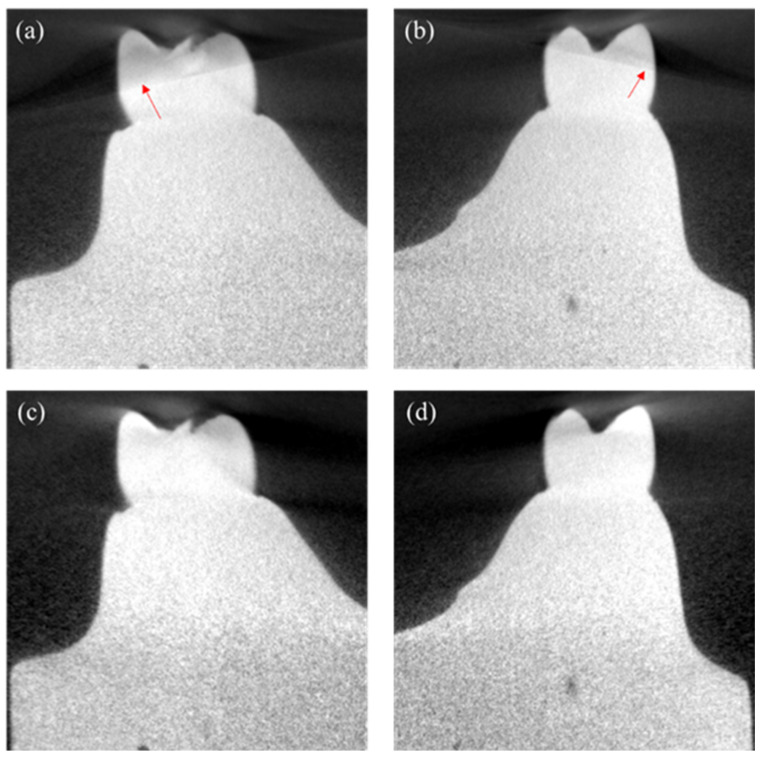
(a,b) show two regions of interest in the images reconstructed by the FDK algorithm. (c,d) show the corresponding regions in the images reconstructed by the CW-FDK algorithm. We can see that the cone-beam artifacts (indicated by the red arrow) have been much reduced in the images of the CW-FDK algorithm.
